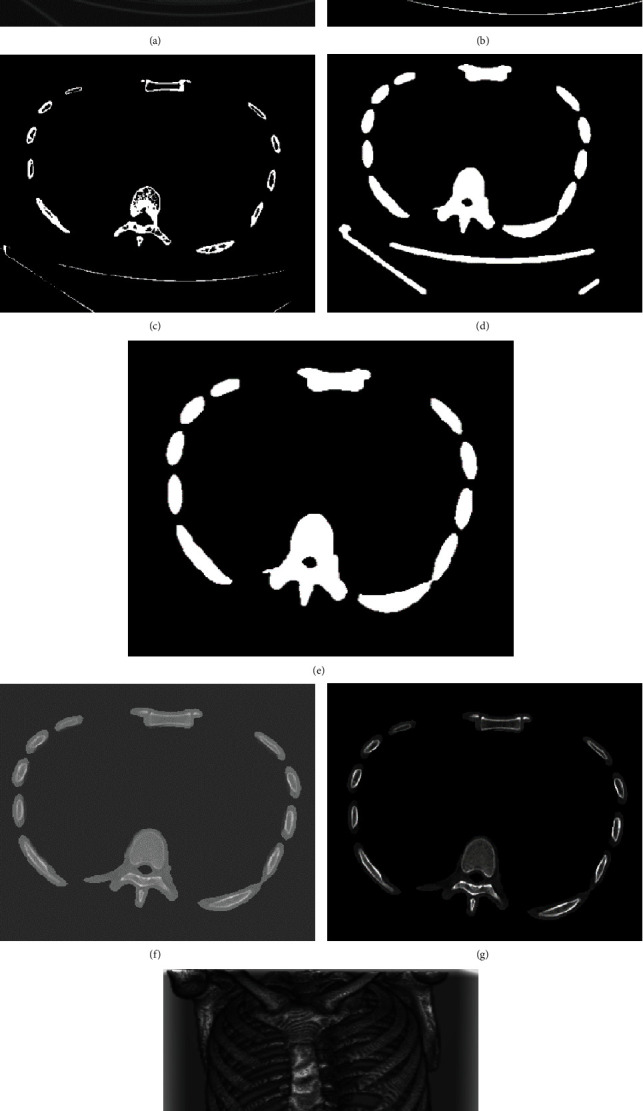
The procedures of preprocessing: (a) the original CT image, (b) the binary bone region mask after thresholding at 180 HU, (c) the mask after removing small connected components, (d) the mask after morphological dilation, (e) the mask after extracting the largest connected component, (f) the extracted bone regions by applying the mask, (g) the bone regions after data normalization, and (h) the 3D view of the preprocessing output.
